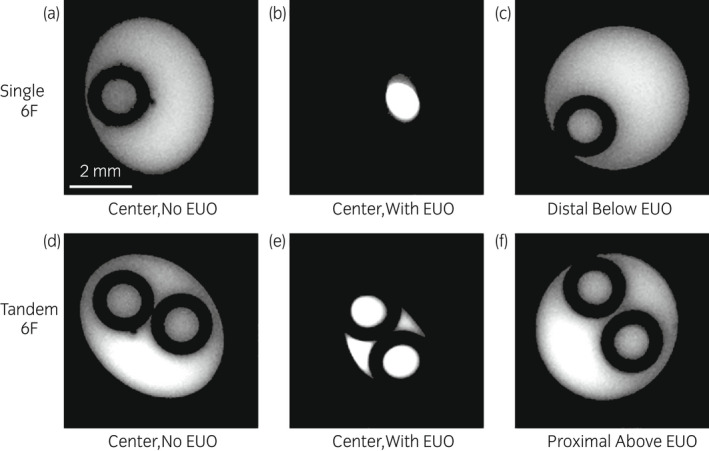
Representative MRI stented ureter cross‐sections showing the stent(s) within the ureter lumen, without and with EUO. Colors: Black represents no flow regions (stent walls and border of ureter walls); white/gray represents fluid velocity, normalized within each image, with higher intensity of white representing higher velocity. (a) Single 6F, no EUO; (b) single 6F, with EUO; (c) single 6F, distal to the EUO; (d) tandem 6F, no EUO; (e) tandem 6F, with EUO; and (f) tandem 6F, proximal to the EUO. [Colour figure can be viewed at wileyonlinelibrary.com]
